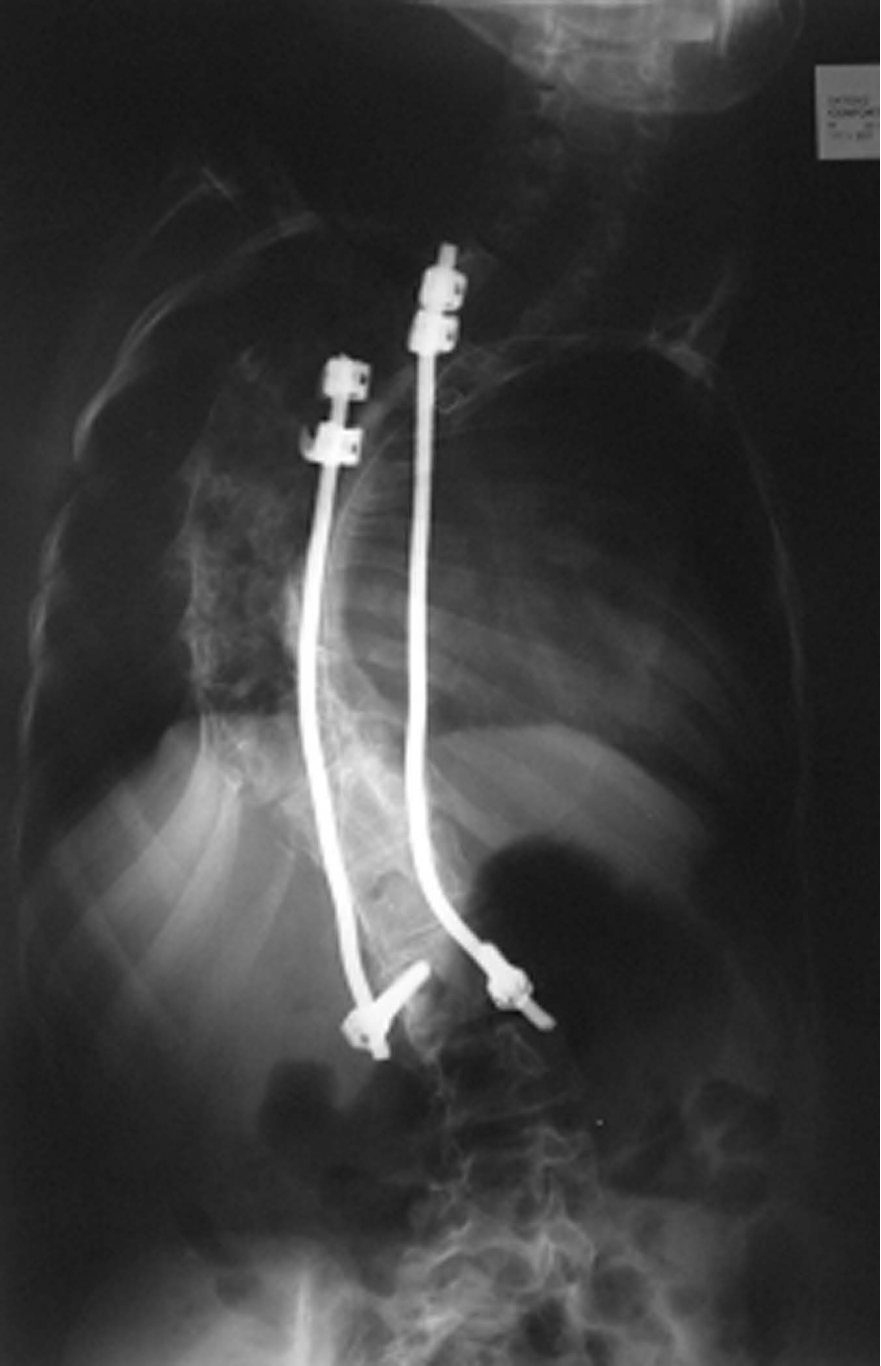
Case 4: Spine radiographs at a 4-year follow-up after inserting the new Dual Rod instrumentation; anteroposterior view.
